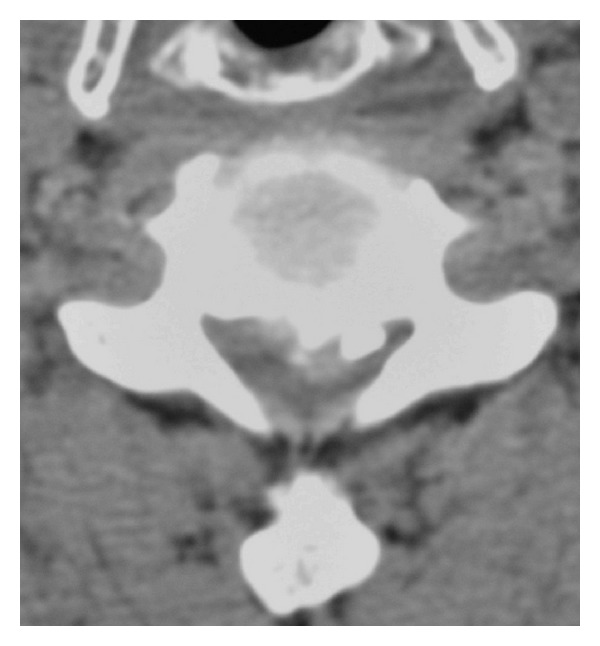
CT-scan transverse cut at the C5-6 level. Segmental type ossified posterior longitudinal ligament on the left side, with adjacent soft tissue mass protruding into the spinal canal.
